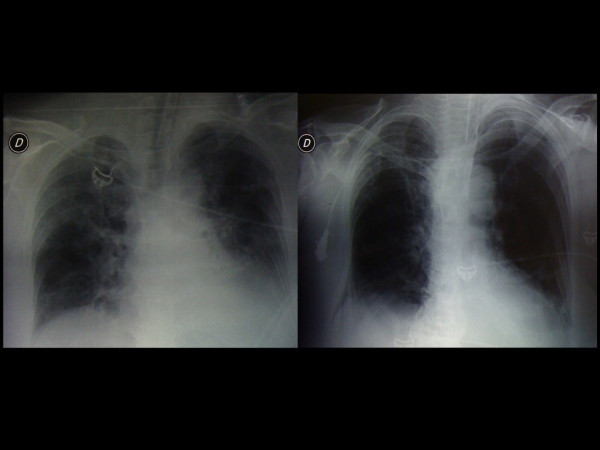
Right panel: antero-posterior chest x-ray of patient 1. Left panel: antero-posterior chest x-ray of patient 2.
